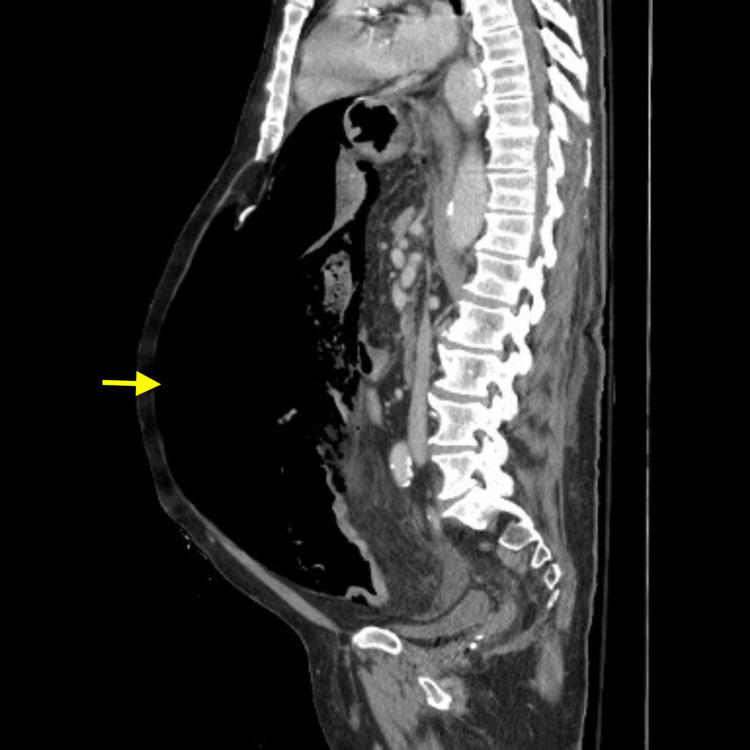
Abdominal/pelvic CT with intravenous contrast, sagittal section demonstrating pneumoperitoneum CT, Computed Tomography
